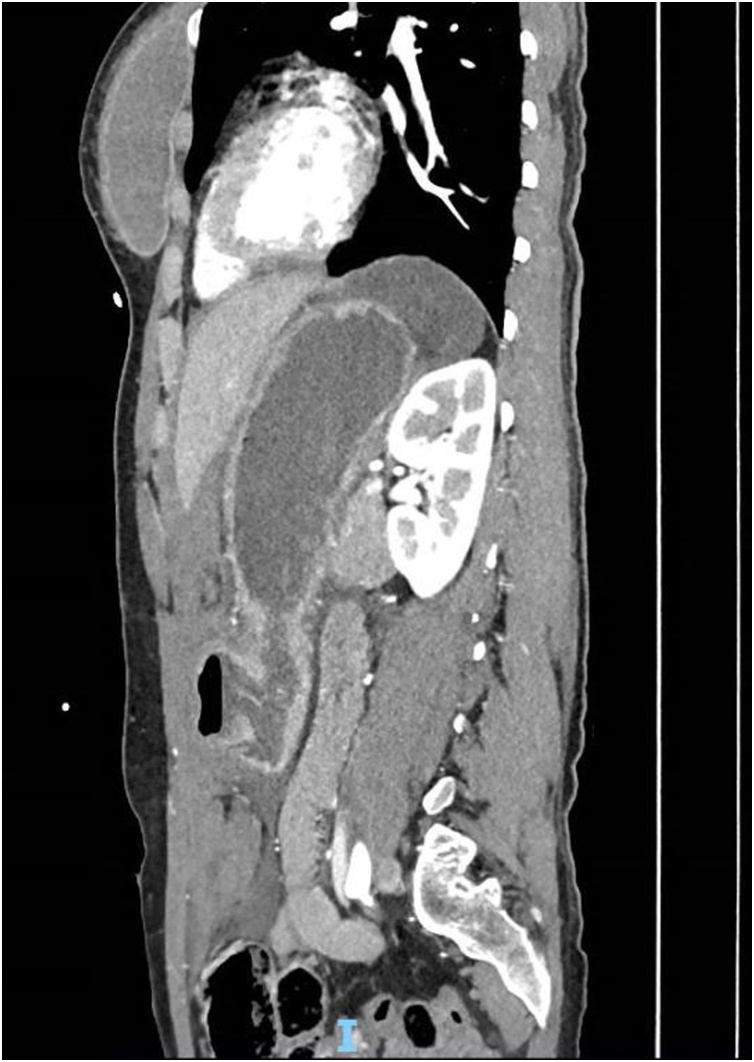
Sagittal CT demonstrating pyloric and duodenal discontinuity with small volume free air just beyond the pylorus.
